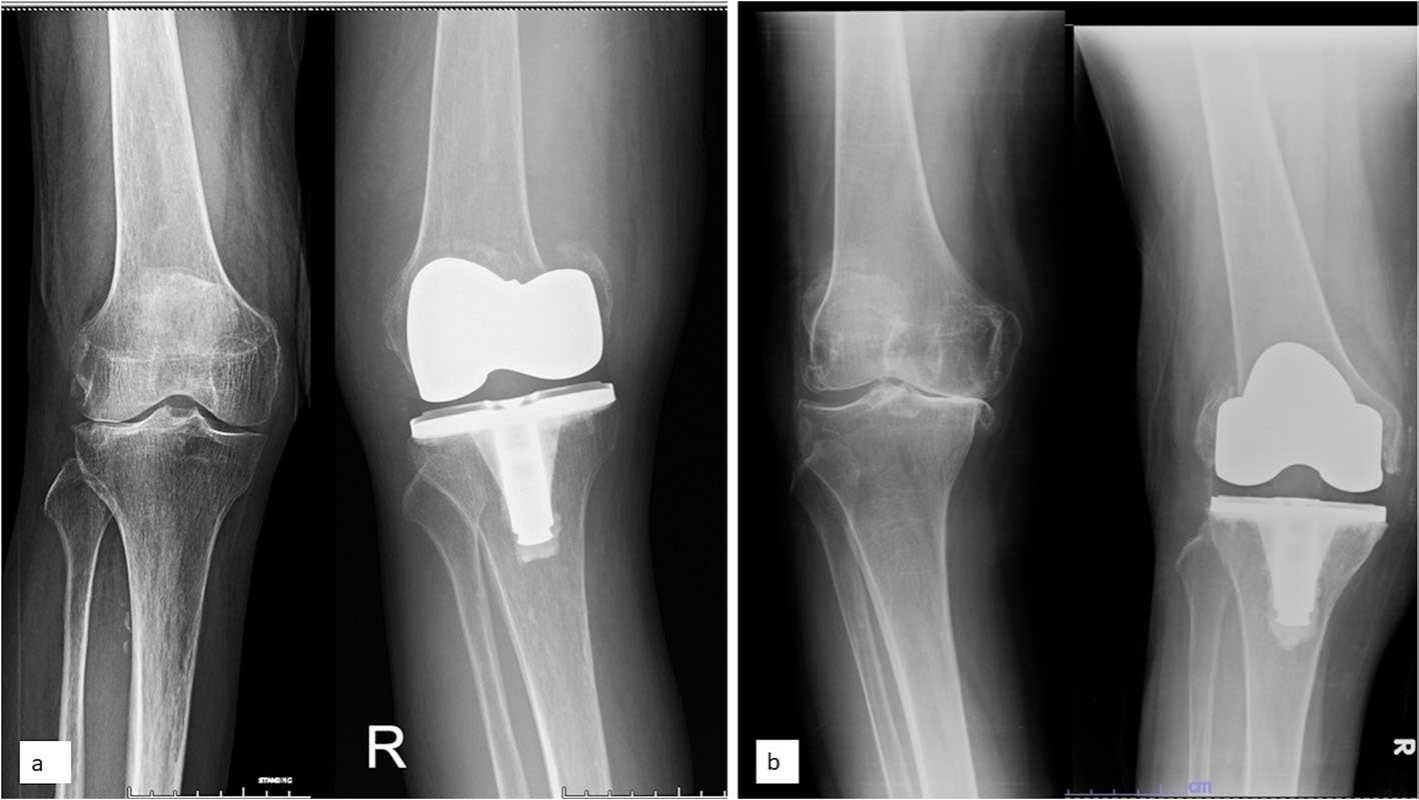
Preoperative and postoperative anteroposterior knee radiographs of two patients demonstrating a) medial condylar fracture and b) lateral condylar fracture, respectively
